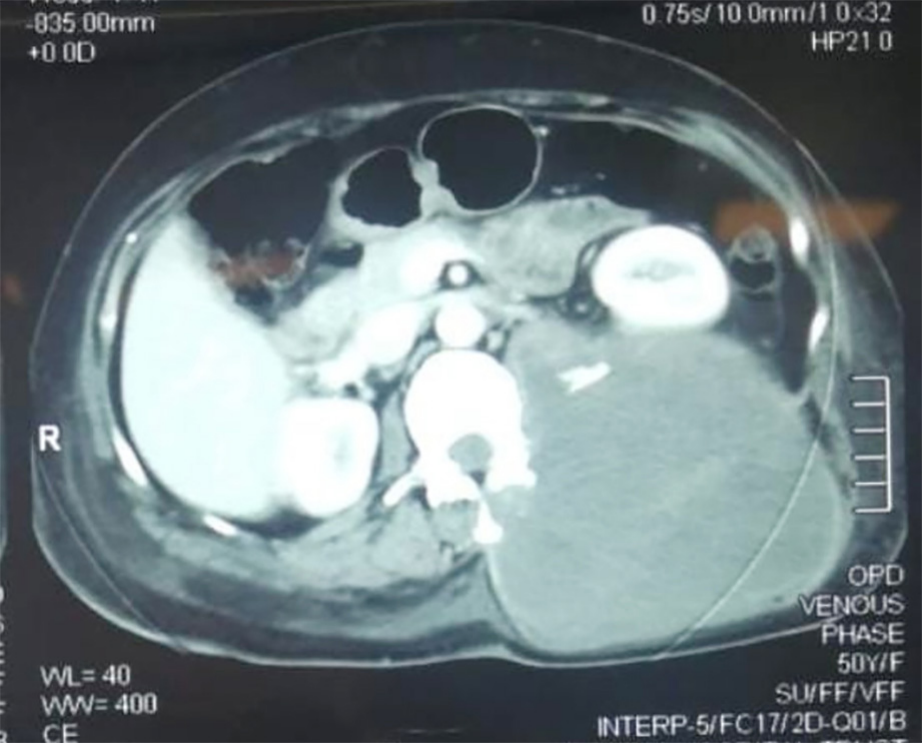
MRI with contrast showing large soft tissue mass measuring 99 × 96 mm in the left posterior paravertebral compartments at the lower lumbar region (yellow arrow).
